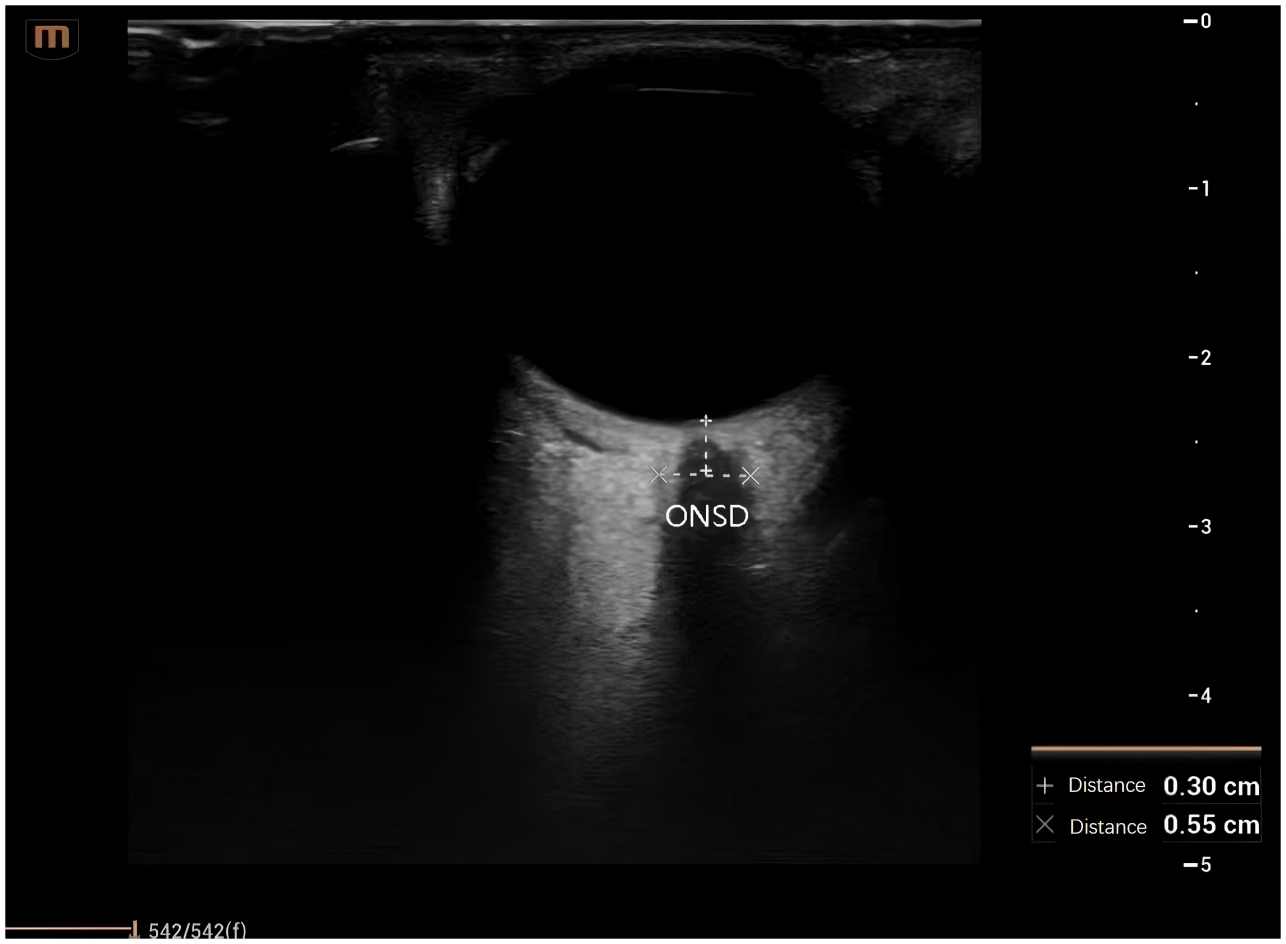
Ultrasound assessment and measurement of the optic nerve. ONSD, optic nerve sheath diameter.
